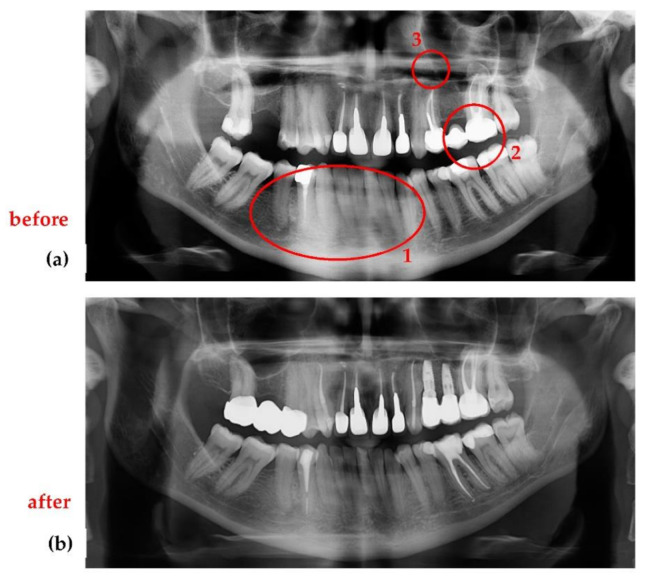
Panoramic radiographs performed on the same patient (L.C., female, 42 years old) (a) before and (b) after the optimization.
